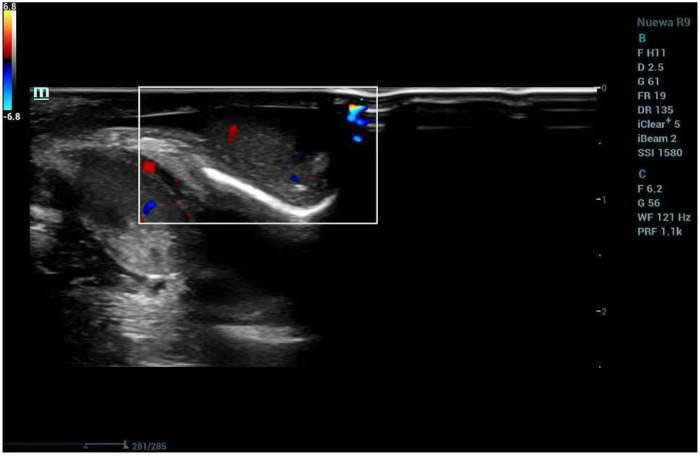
The results of testicular ultrasound in the proband.
